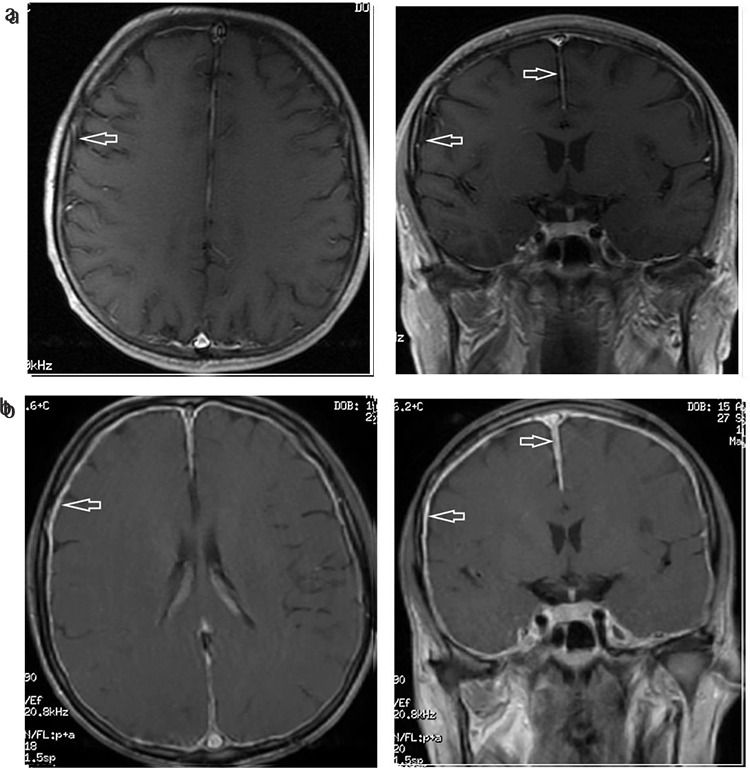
Cerebral MRI revealed leptomeningeal involvement (a), but there was no evidence of meningeal involvement upon cerebral MRI after 2 months (b).
